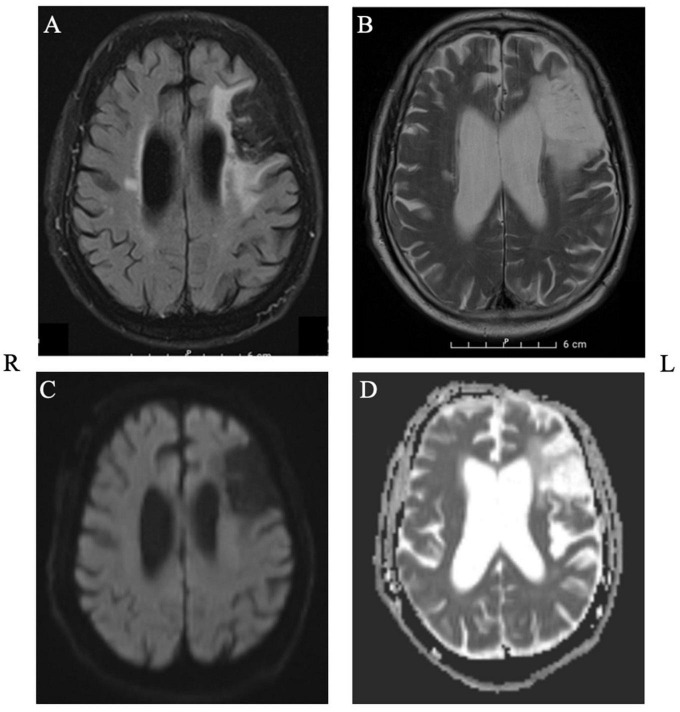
Structural MRI depicting the extent of the lesion in axial plane. Panel (A): T2_FLAIR, Panel (B): T2_TSE, Panel (C): DWI, Panel (D): ADC.
